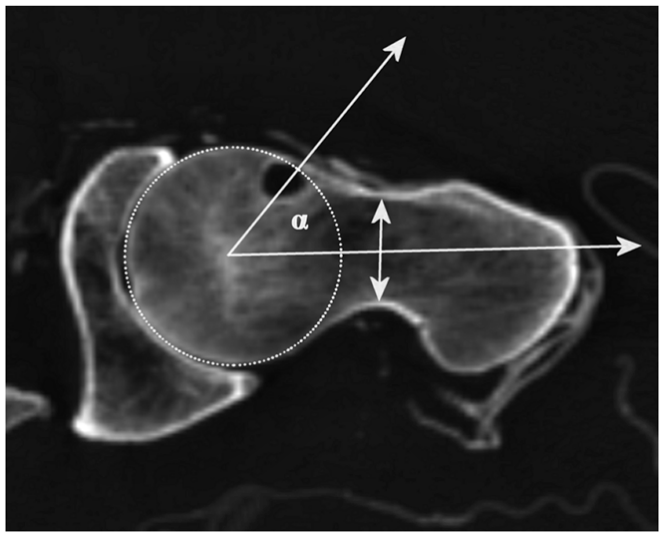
Measurement of the angle α. Paraaxial (parallel to the axis of the femoral neck) reformation CT image in case 3. The angle α was defined by a line between the centre of the femoral head and the anterior point, where the distance of the centre of the femoral head exceeds the radius of the surface of the femoral head and a second line between the centre of the femoral head and the centre of the femoral neck at its narrowest point.
